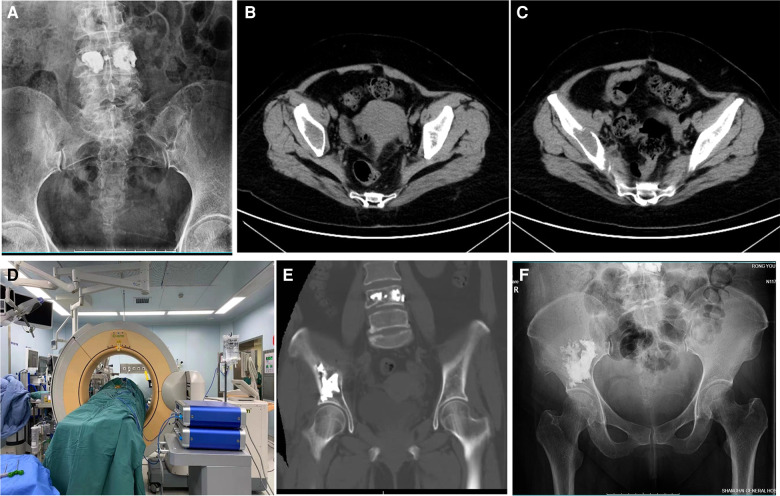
59-year-old man with metastatic liver carcinoma and a painful pelvic metastasis. (A) Preoperative pelvic x-ray. (B,C) Preoperative pelvic CT scan. (D) Intraoperative O-arm 3D image during puncture and cementoplasty. (E) Postoperative pelvic CT scan. (F) Postoperative pelvic x-ray 6 months after the procedure.
